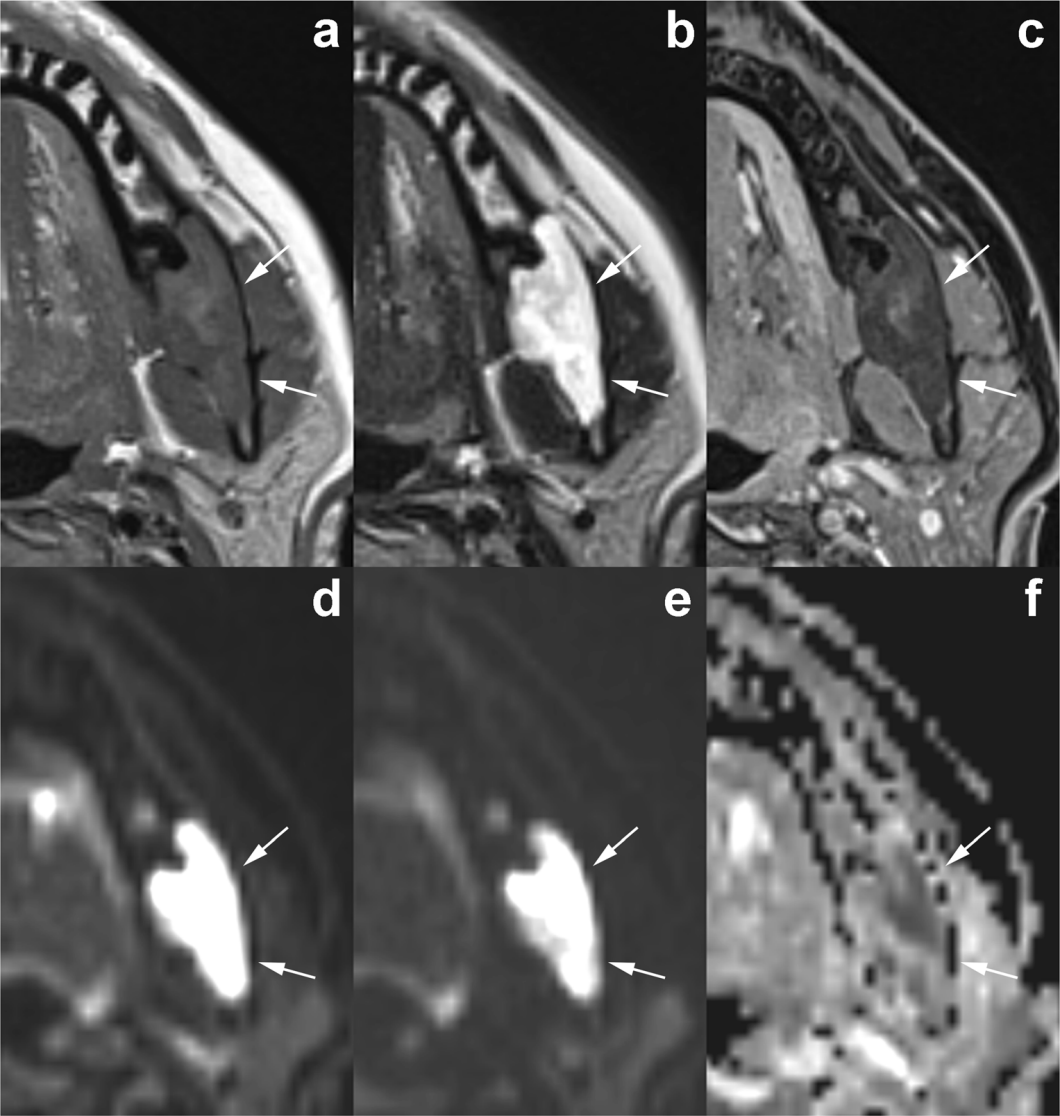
Axial magnetic resonance imaging (MRI) images demonstrate the typical signal pattern of OKC. The lesion, located in the posterior left mandible (*arrows*), shows intermediate-high signal intensity on T1-weighted sequence (a) and heterogenous high signal intensity on T2-weighted sequence (b). No enhancement is observed within the lesion on enhanced T1-weighted fat-saturated sequence (c). Diffusion-weighted imaging demonstrates restricted diffusion with high signal on b0 (d) and b1000 (e) images and low signal intensity on apparent diffusion coefficient (ADC) map (f)
